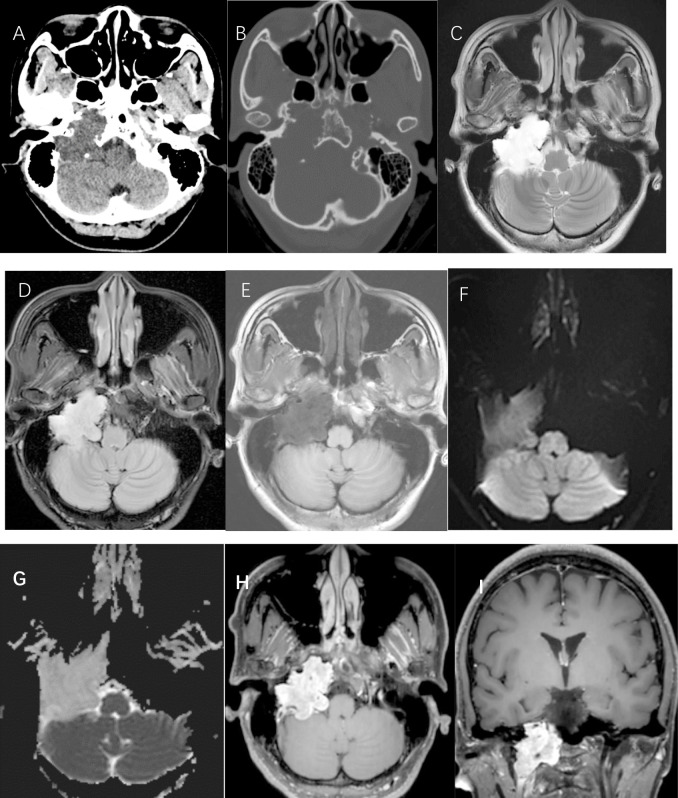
CT scans and MRI. (A, B) CT-scans. (C-I) MR images: (C) axial T2-weighted sequence; (D) axial T2-weighted fluid-attenuated inversion recovery sequence; (E) axial T1-weighted sequence; (F) diffusion-weighted imaging; (G) apparent diffusion coefficient imaging; and (H, I) T1-weighted gadolinium-enhanced sequence.
